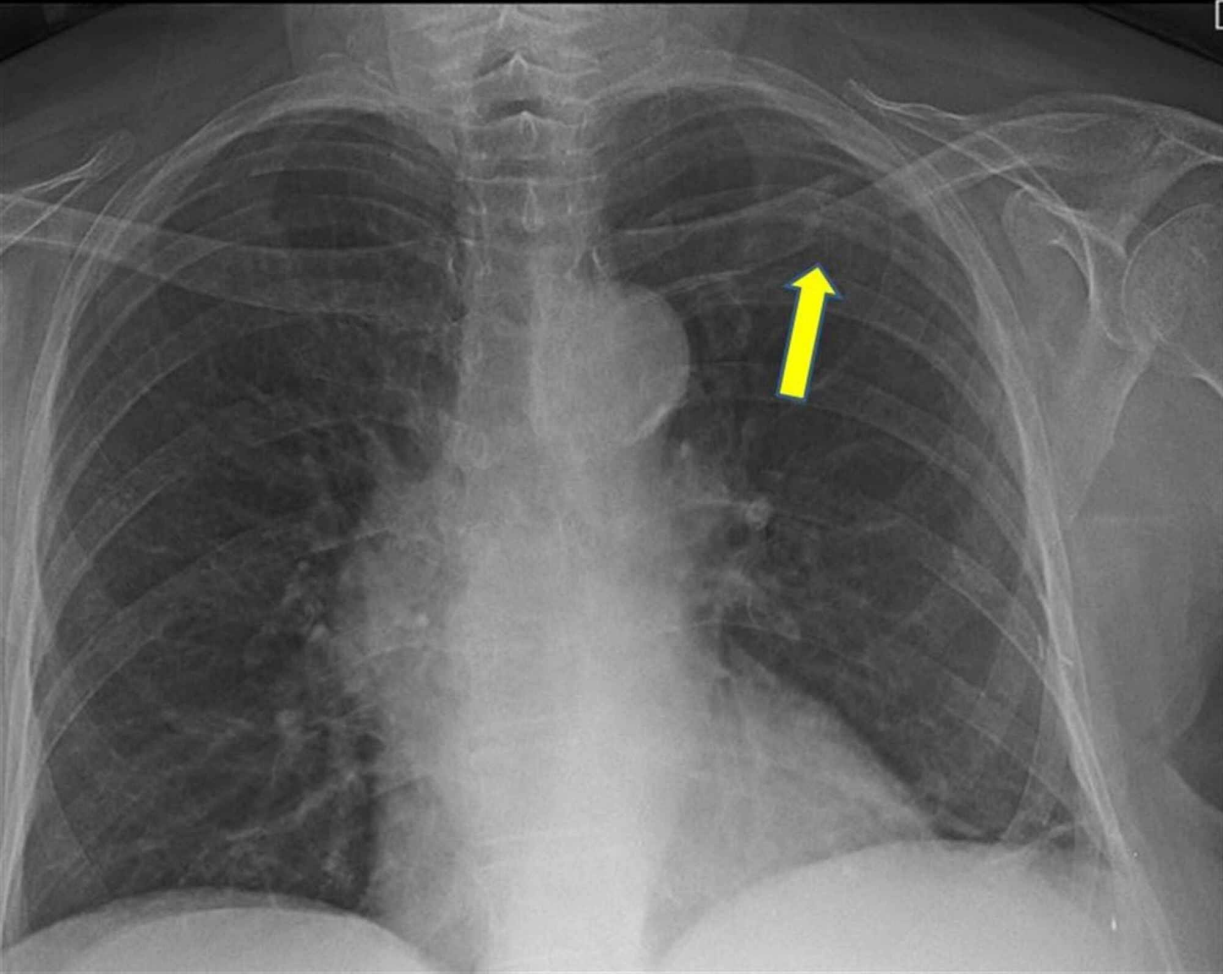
Fracture of the left clavicle in the X-ray
